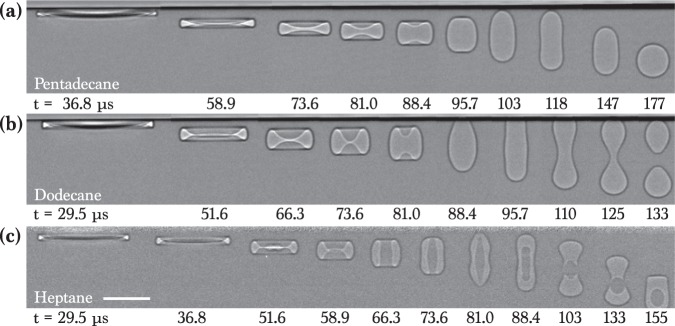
X-ray images of air evolution for oils. (a) Impact of the pentedecane drop (*μ* = 2.8 mPa s) at *H* = 8 cm, indicating one bubble. (b) Impact of the dodecane drop (*μ* = 1.5 mPa s) at *H* = 8 cm, indicating a double bubble. (c) Impact of the heptane drop (*μ* = 0.4 mPa s) at *H* = 16 cm, indicating an antibubble. All individual images were taken with one X-ray pulse of 472 ns long. All the images were background-corrected. The time at impact moment was set to *t* = 0. The scale bar is 100 *μ*m long.
